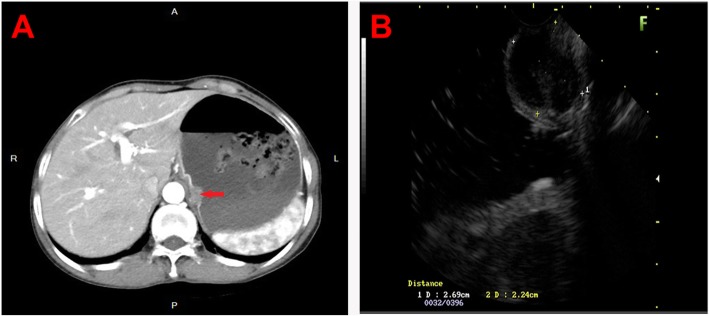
Images of the case with CT and EUS. a, Contrast-enhanced abdominal CT revealed a 2.0-cm relatively well-defined soft tissue mass (red arrow) on the small curved side of the cardia; b, Linear EUS examination revealed a hypoechoic lesion that originated from the fourth layer, with an uneven internal echo and a clear boundary
